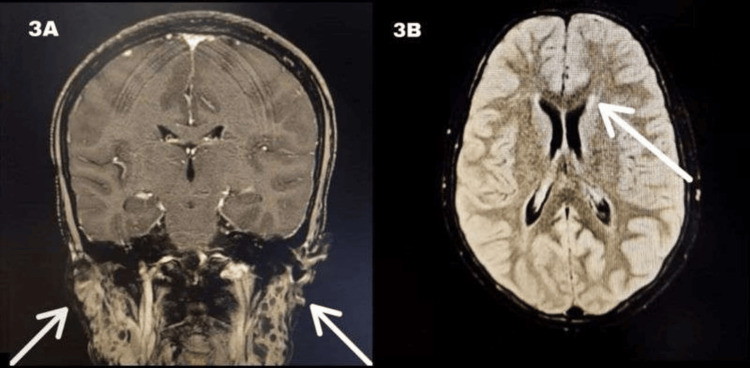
(A) bilateral parotid enlargement and (B) hyperdense foci on post-contrast MRI These image findings are indicative of benign lymphoepithelial lesions, a salivary gland disease seen commonly in HIV positive individuals. MRI: magnetic resonance imaging, HIV: human immunodeficiency virus
